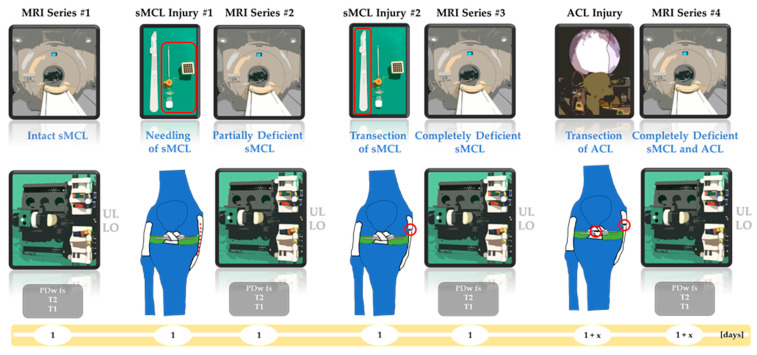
Timeline of MRI series and graded injuries. Sequentially, the superficial medial collateral ligament (sMCL) was partially injured, i.e., needled (sMCL injury #1), and completely injured, i.e., transected (sMCL injury #2). The anterior cruciate ligament (ACL) was transected (ACL injury). Consequently, the knee joints were imaged in the sMCL-intact condition (MRI series #1), partially sMCL-deficient condition (MRI series #2), completely sMCL-deficient condition (MRI series #3), and the combined sMCL- and ACL-deficient condition (MRI series #4). MRI series refer to the imaging protocol (grey boxes) that was completed for each joint in the unloaded (UL) and loaded (LO) configurations. Unit of timeline is days (yellow).
